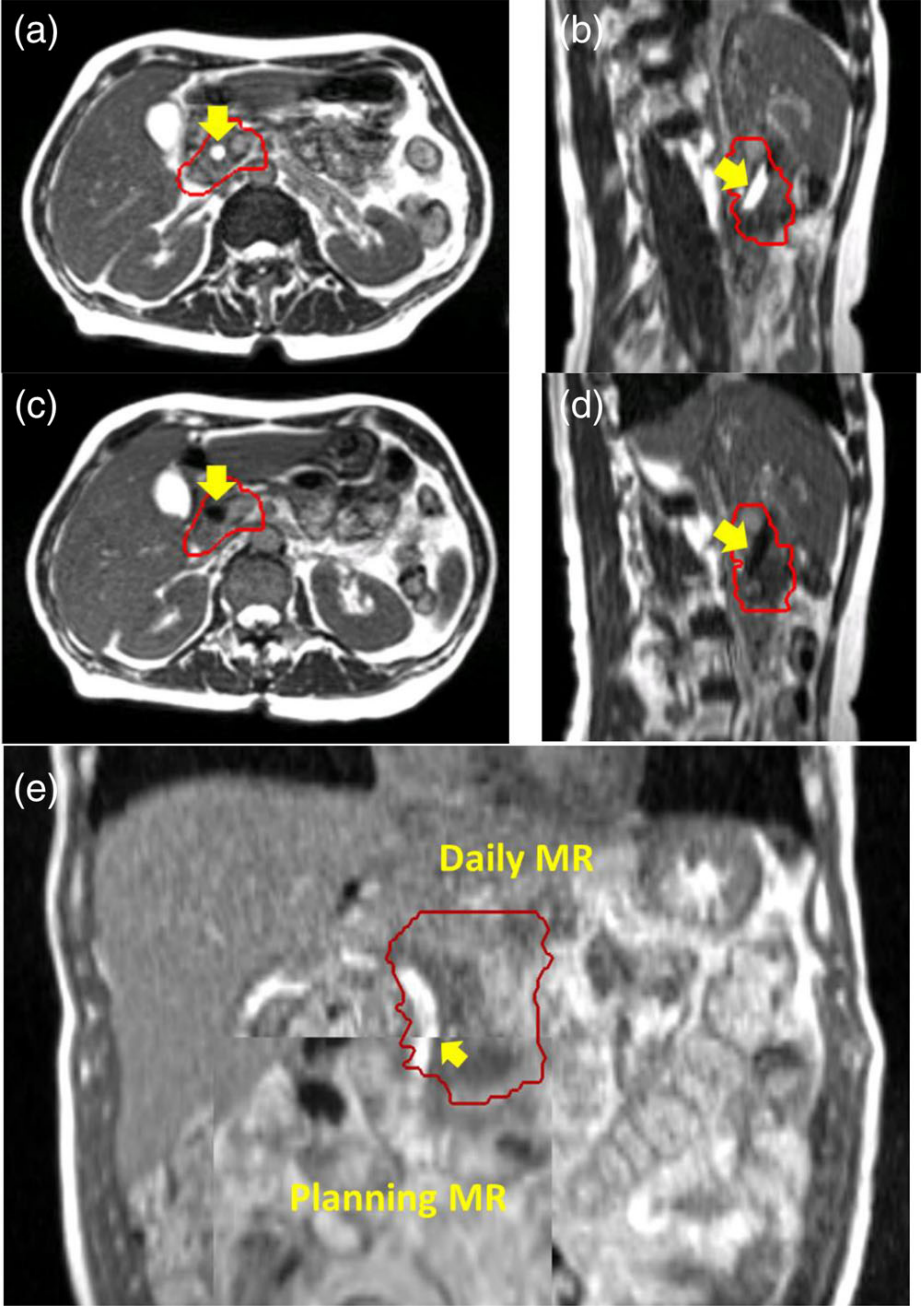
The appearance of the biliary stent (arrows) varied on TrueFISP MR images depending on daily stent filling. It appeared bright when filled with liquid (A and B) and dark when filled with air (C and D). When registered with soft tissue online, the stent can be misaligned (arrow in E). Red contours show the PTV.
